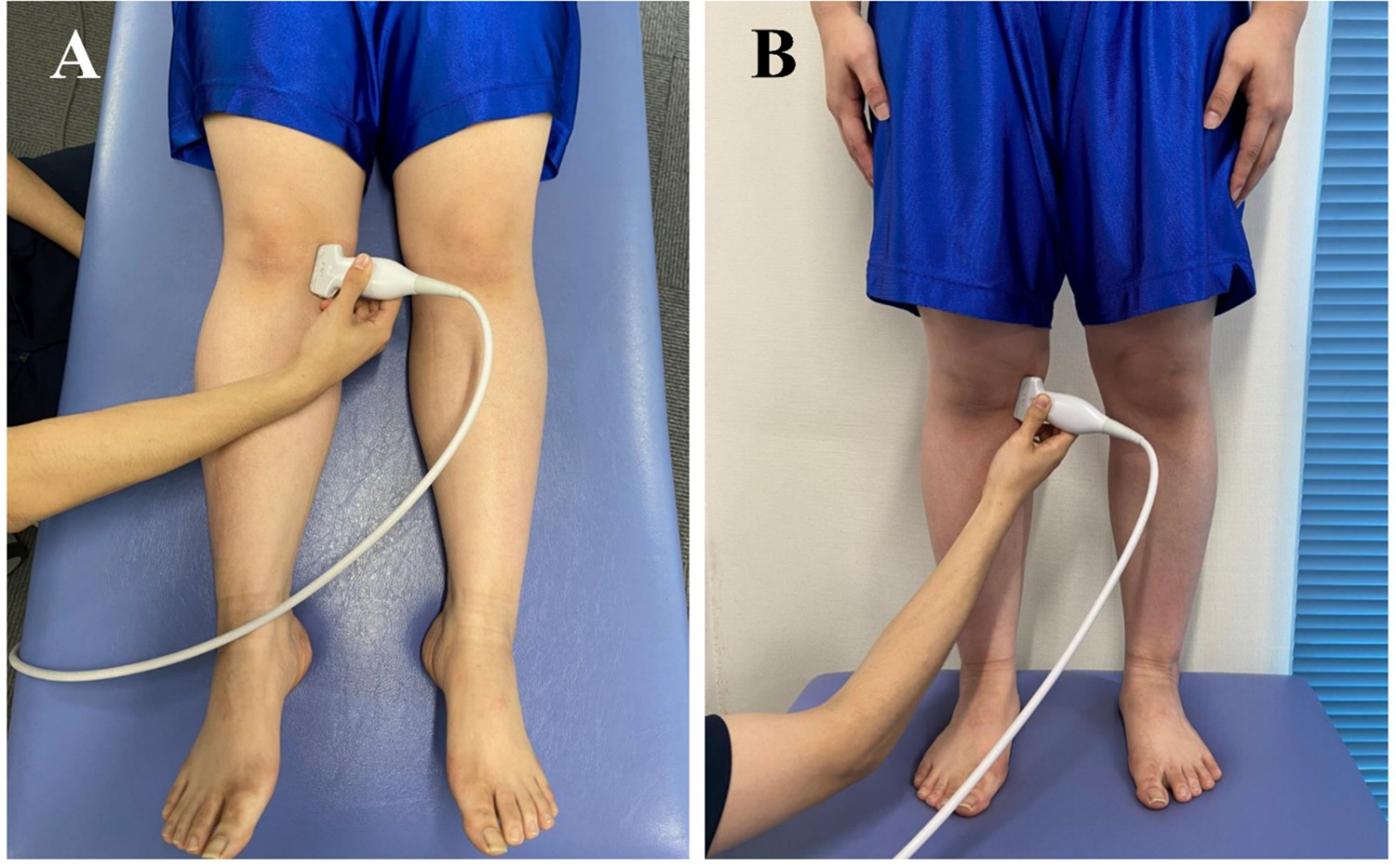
Measurement of the medial knee joint using ultrasound. Ultrasound assessed the medial meniscus when the patient was in the supine (A) and standing (B) positions. Both measurements were performed at maximum knee joint extension in each patient. The transducer was placed longitudinally at the level of the medial collateral ligament at the medial aspect of the knee joint.
